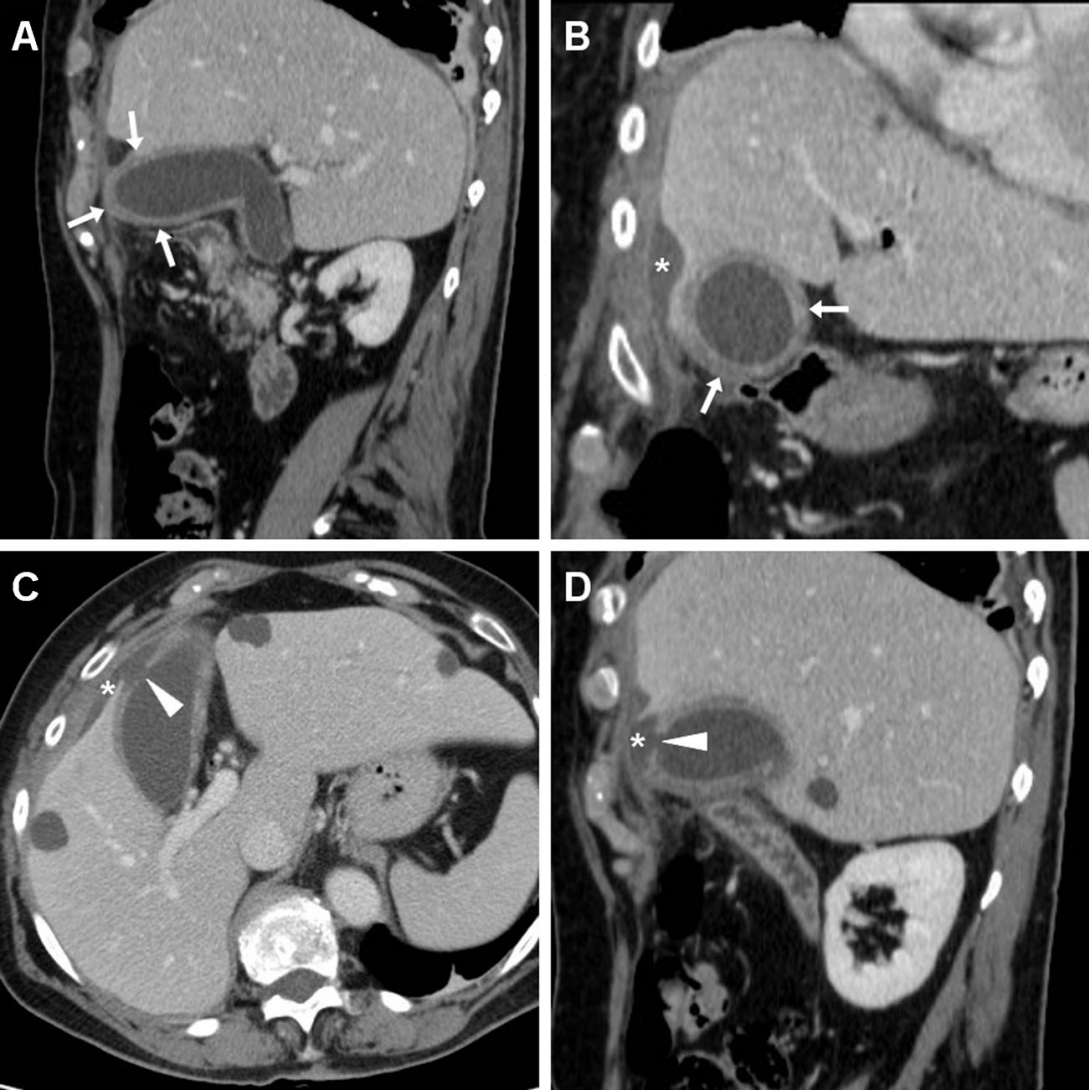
Acute cholecystitis in a 66-year-old female manifesting with fever and transverse upper abdominal pain 3 days after ERCP with sphincterotomy and biopsy of ampullary adenoma including antibiotic prophylaxis. Multiplanar contrast-enhanced CT images show a thickened gallbladder wall (*arrows*) with focal perforation (*arrowhead* in c) and localised collection (*), which required emergency laparotomic cholecystectomy.
